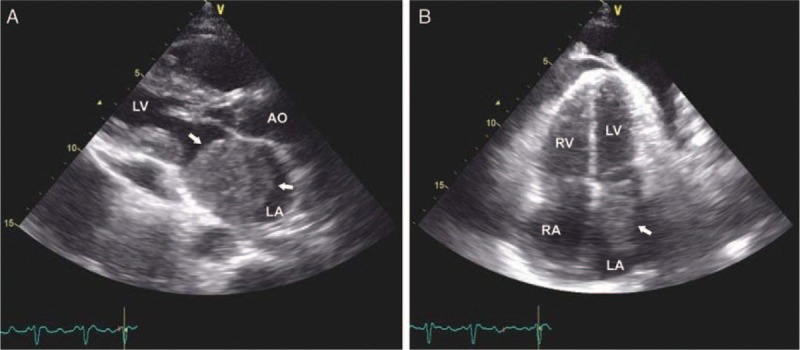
Transthoracic echocardiography. Transthoracic echocardiography in (A) parasternal long-axis and (B) apical 4 chamber views show a mass occupying the left atrium (arrows) and small amount pericardial effusion. AO = aortic root, LA = left atrium, LV = left ventricle, RA = right atrium, RV = right ventricle.
